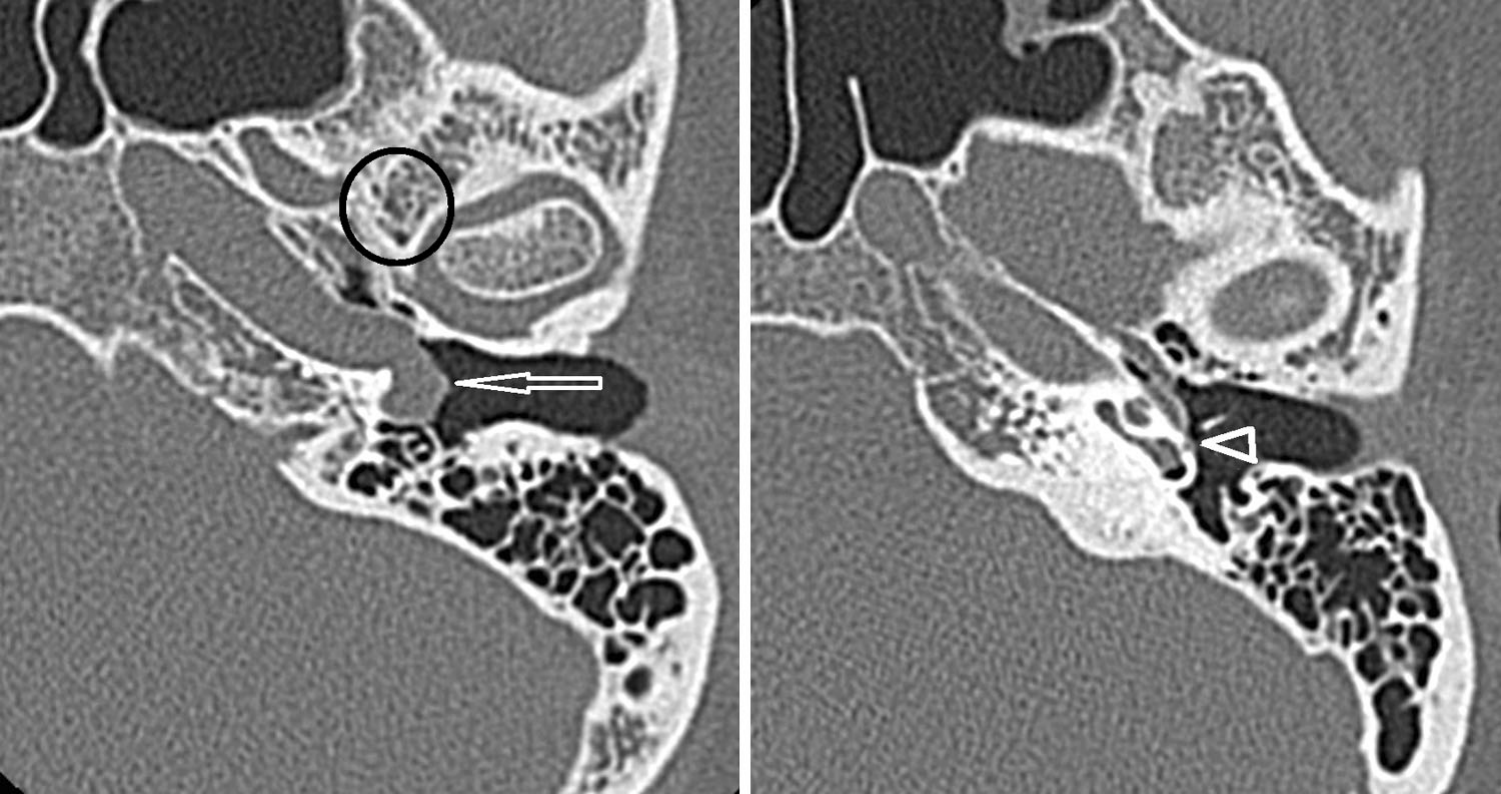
Aberrant course of the internal carotid artery (*arrow*) and persistence of the stapedial artery (*arrowhead*) on thin-sliced CT. Note the absence of the foramen spinosum (*encircled*)
